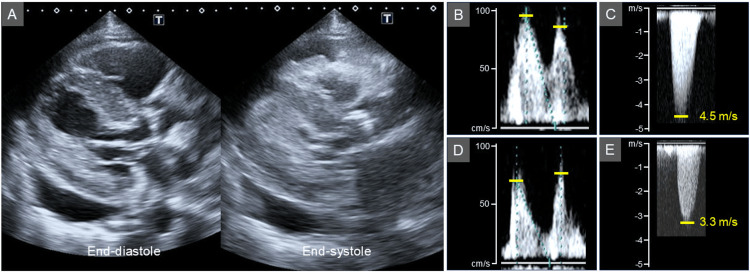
Transthoracic echocardiographic images in case 1. Transthoracic echocardiography showed a high ejection fraction (EF) of 80% (A), left ventricular hypertrophy, a calcified aortic valve, an early to late diastolic filling velocity ratio (E/A) of 1.2 (B), and pericardial effusion. The peak aortic jet velocity was 4.5 m/s (C). After thiamine supplementation, EF decreased to 50%, with a change in the E/A to 0.9 (D), and a reduction in peak aortic jet velocity to 3.3 m/s (E).
